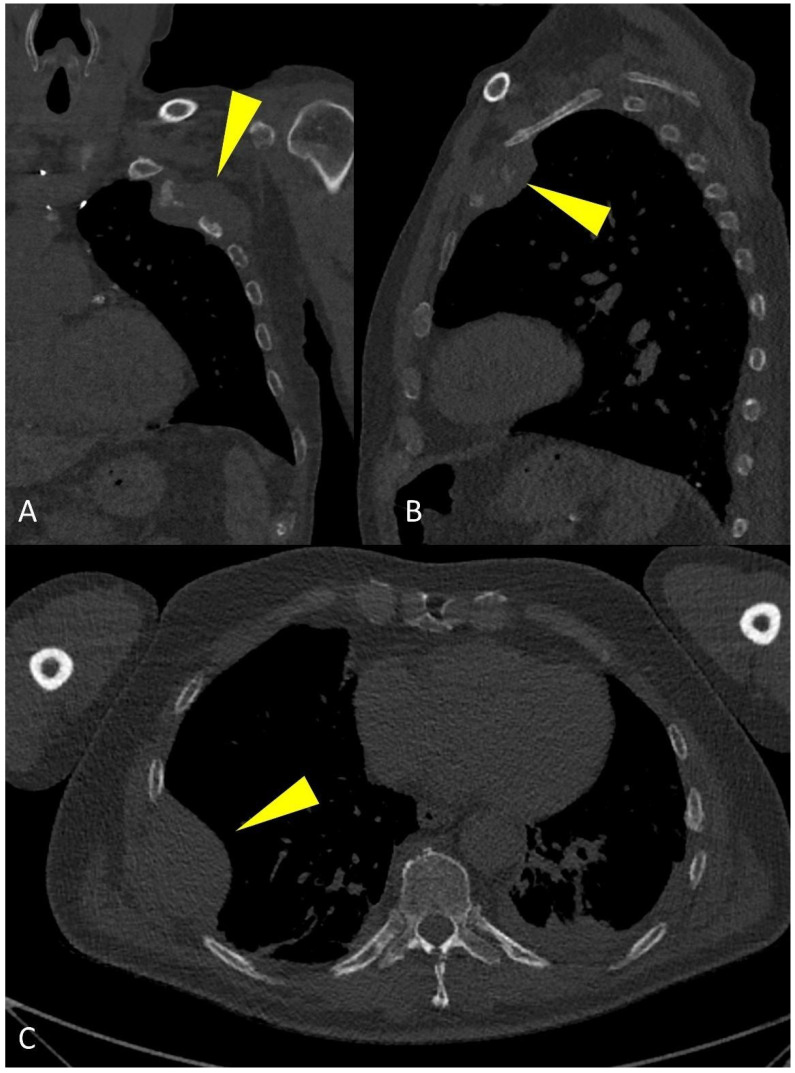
Multiplanar CT images showing a large para-medullary lesion (yellow arrowheads in (A–C)): extra-osseous pathologic tissue in the chest wall soft tissues originating linked to skeletal involvement of the rib.
